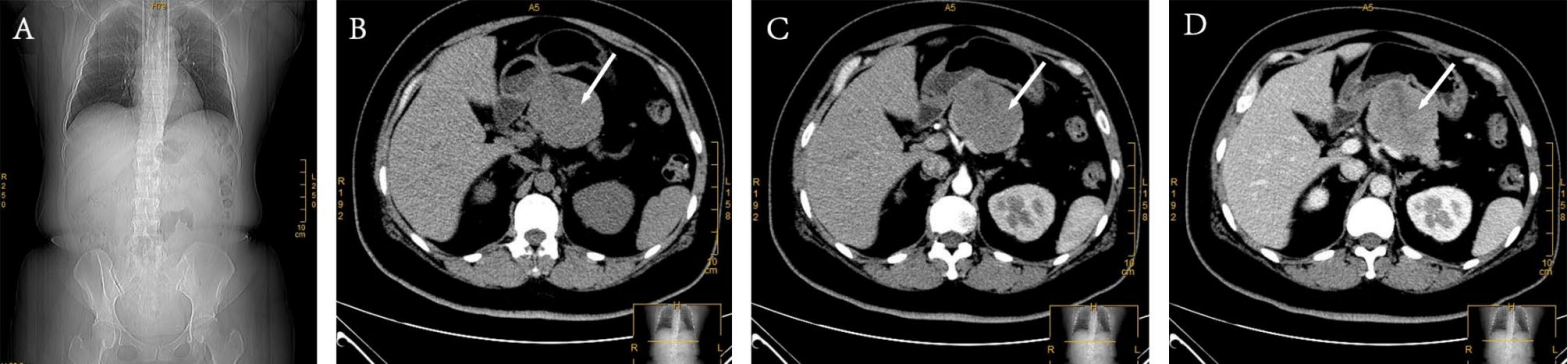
Preoperative abdominal CT. A: Abdominal x-ray plain films; B: Non-contrast enhanced CT; C: Arterial phase of contrast-enhanced CT; D: Venous phase of contrast-enhanced CT; Note: The tumor indicated by the arrow in the figure is a pancreatic schwannoma. It is a low-density solid mass under non-contrast enhanced CT, and heterogeneous enhancement can be seen under contrast-enhanced CT
